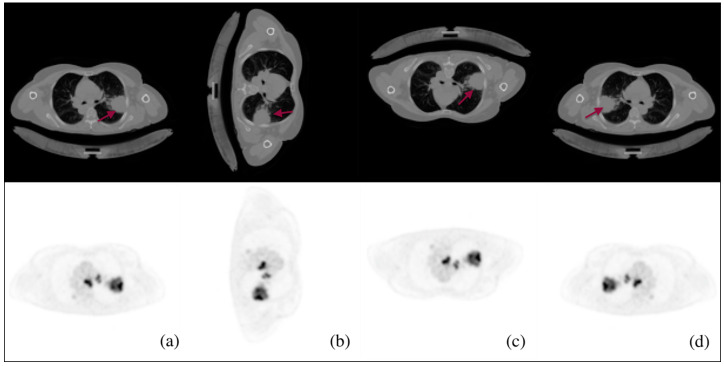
Some examples of the augmentation process of CT and PET images for STS: (a) the main CT-PET, (b) rotating the CT-PET by 90 degrees clockwise, (c) flipping the CT-PET upside down, and (d) left-mirroring the CT-PET. Red arrows indicate the tumour region.
